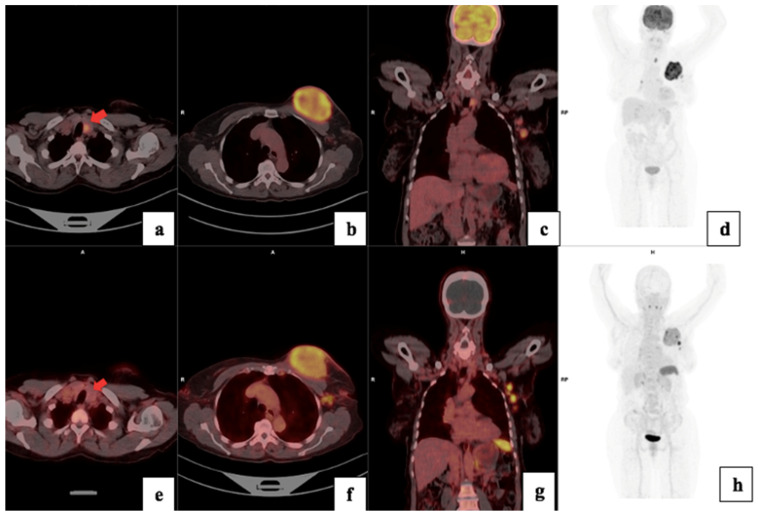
^18^F-FDG (a–d) and ^68^Ga-Pentixafor (e–h) images showing increased uptake in the left breast primary (b,f) and left axillary lymph nodes (c,g) in a a 64-year-old female with T3N1M0 triple-negative invasive ductal carcinoma of the left breast. The primary tumour exhibited a Ki67 index of 90% and an SUVmax of 7.26, on ^68^Ga-Pentixafor and 20.79 on ^18^F-FDG. Notably, ^18^F-FDG showed high uptake in a benign thyroid lesion (a), which was negative using Pentixafor as indicated by the arrows (e).
